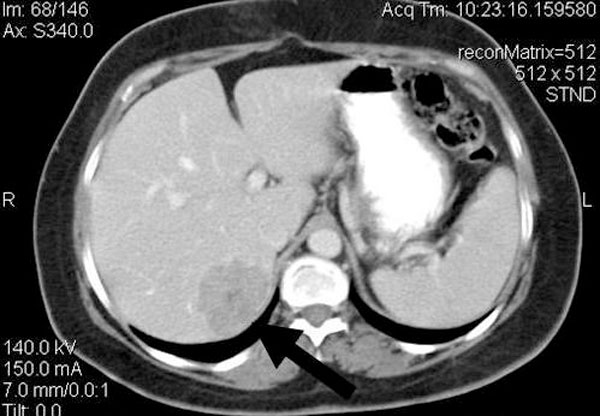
Abdominal CT scan showing a metastatic lesion in the right hepatic lobe. (max diam 2.6 cm).
